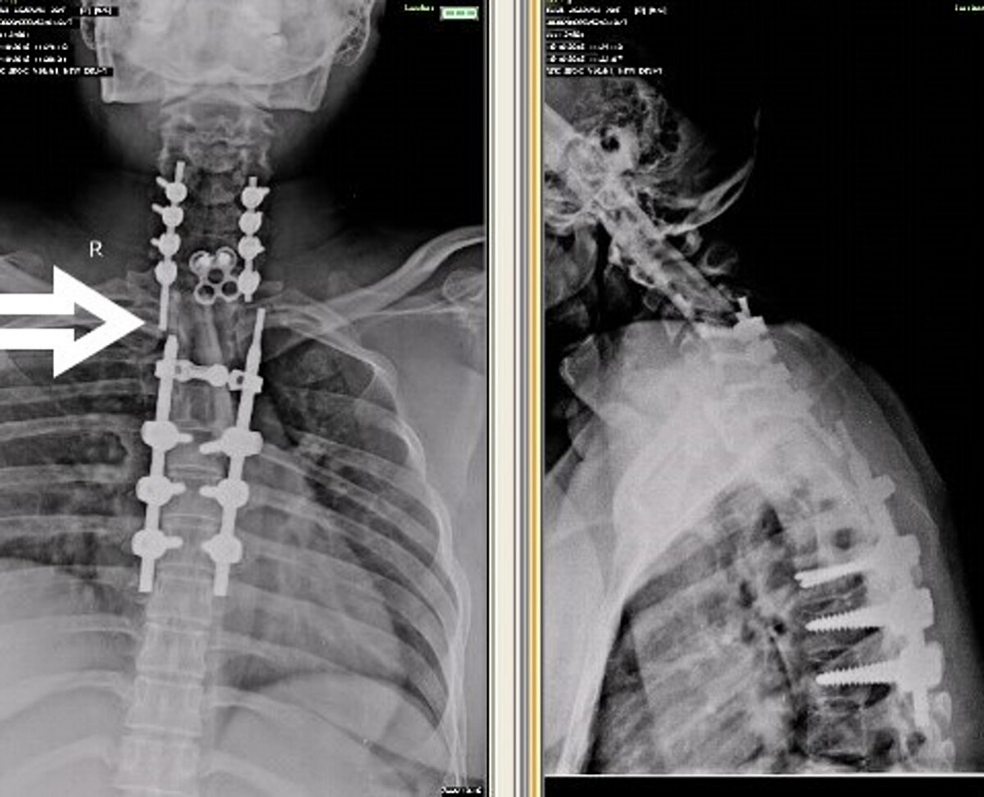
X-ray after four years of surgery showing breakage of both rods
